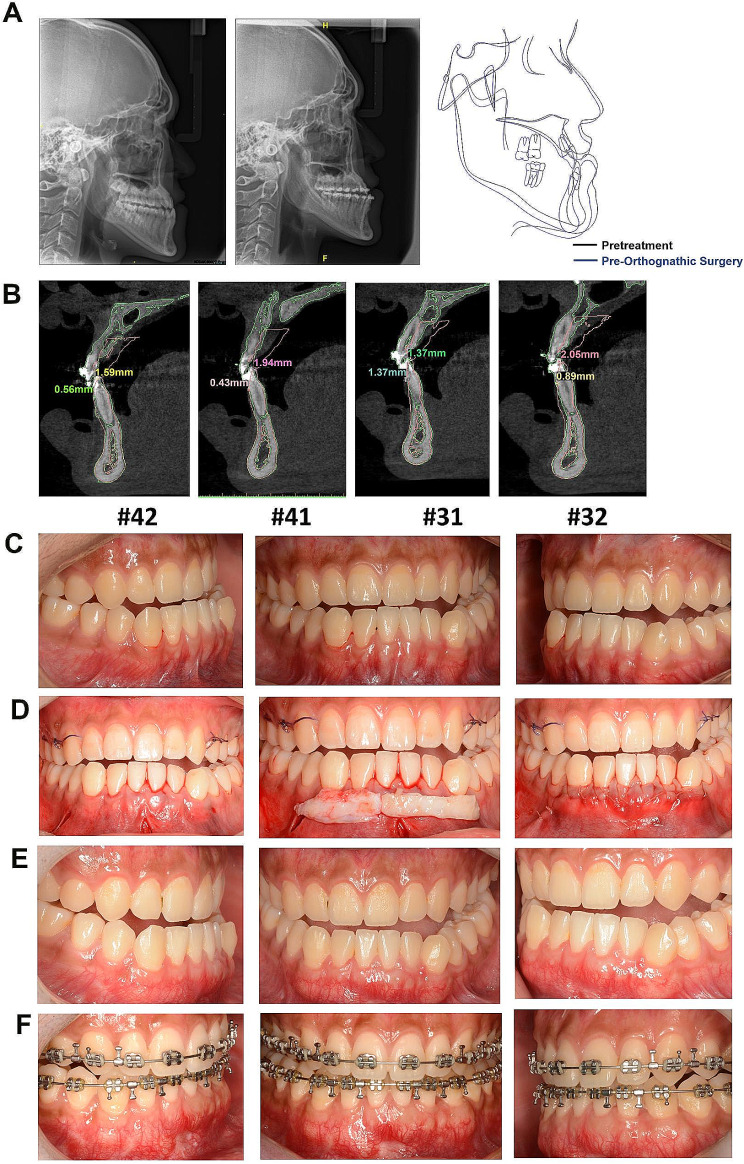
Cephalometric radiographs, CBCT and clinical photographs of case 2. A The comparison of the cephalometric radiographs of the baseline and the end of pre orthognathic surgical orthodontic treatment. B The superimposition images of the #31#32#41#42 CBCT data at baseline and at the end of pre orthognathic surgical orthodontic treatment. C The appearance of the anterior mandibular teeth with thin periodontal phenotype at baseline. D Subperiosteal tunnel was extended from the vestibular to the gingival margin, which accommodated a 2 mm thick and at least 5 mm apico-coronal-dimensioned autogenous connective tissue graft. E The photographs at three months after the PhMT-s surgery. F The photographs at the end of orthognathic surgery
